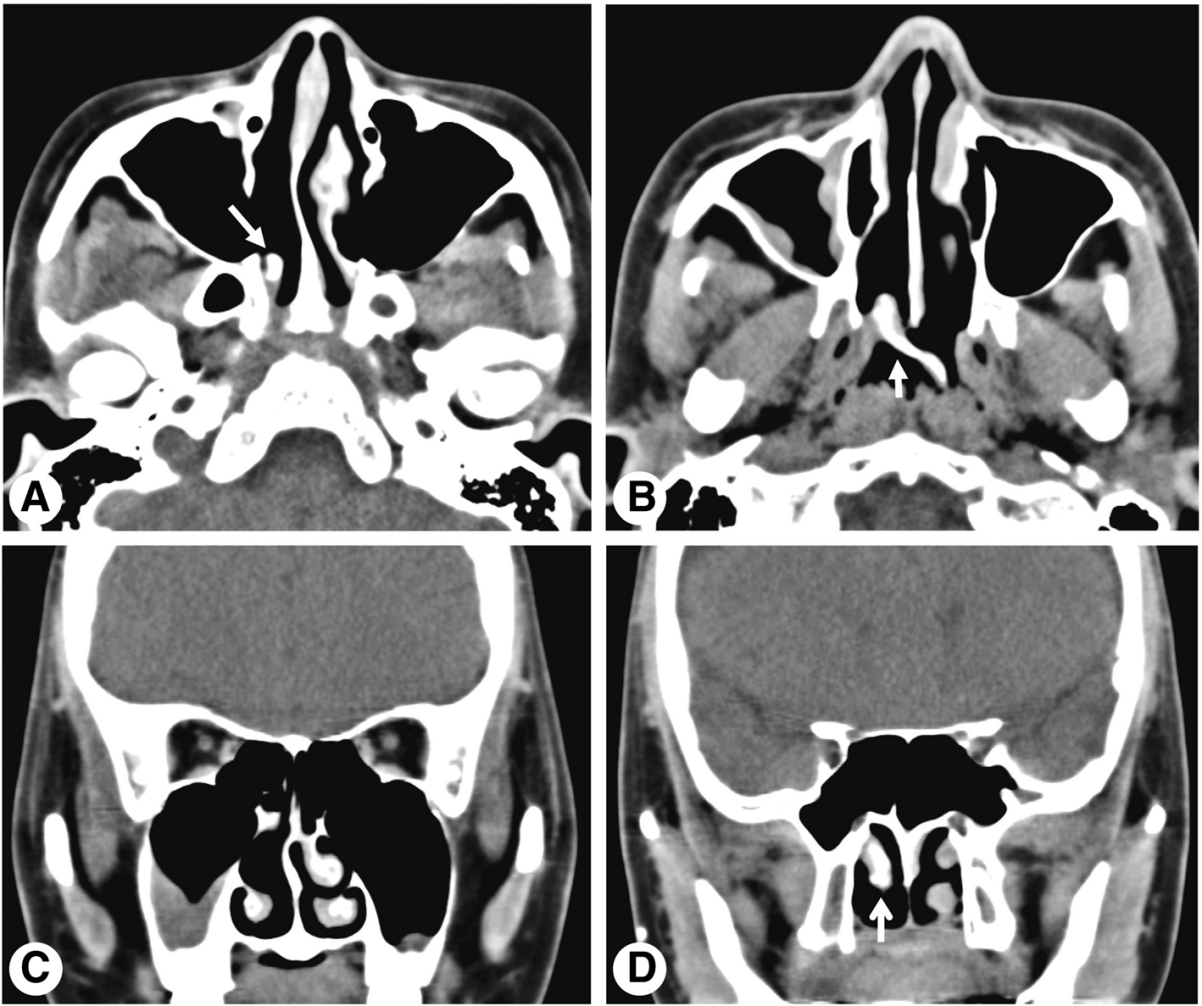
Preoperative computed tomography images of the paranasal sinus. (A, B) Axial computed tomography images show abnormal bony trabeculation (arrow) from the posterior bony attachment of the right middle turbinate to the left posterior nasopharyngeal wall. Coronal computed tomography images show anterior agenesis of the right middle turbinate and right maxillary sinusitis (C), and the right middle turbinate (arrow) extending through the right choana (D).
